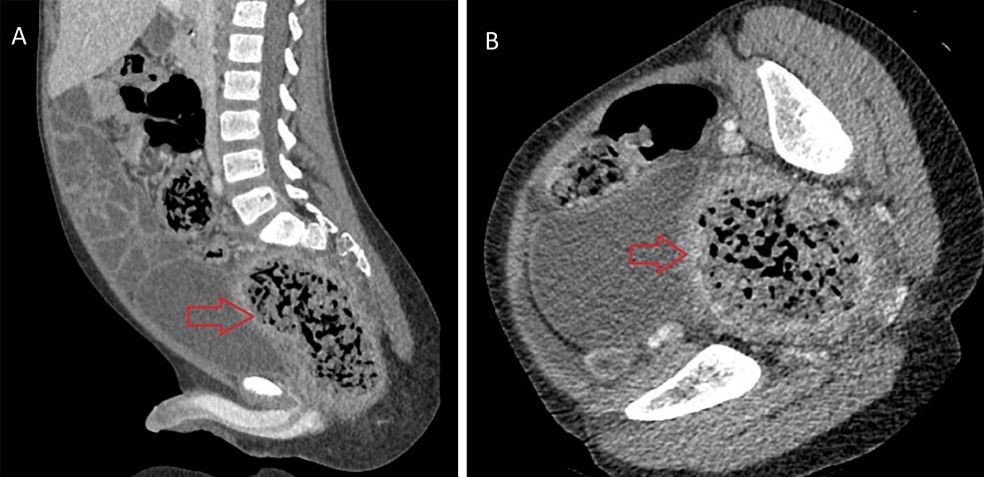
CT images showing large rectal fecalith in rectum measuring 2.6 in (6.6 cm) with mild circumferential rectal wall thickening and enhancement, with perirectal and presacral fat stranding consistent with stercoral colitis; key findings are marked by the red arrows
